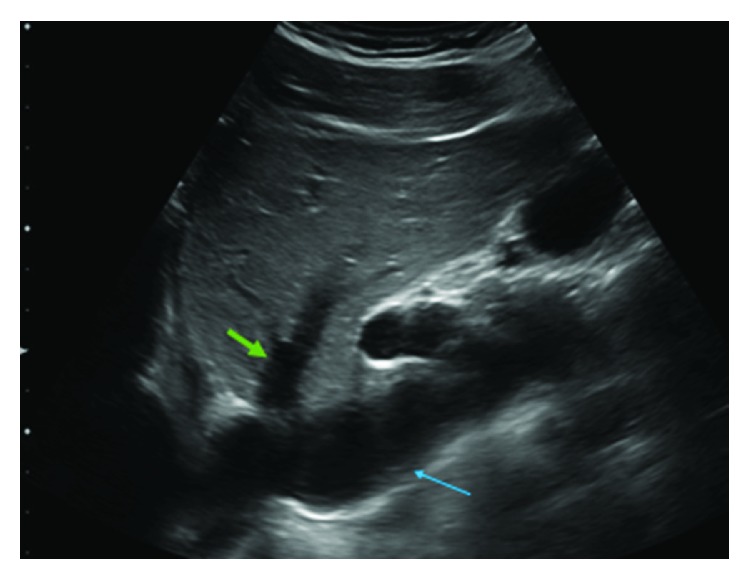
IVC on right upper quadrant ultrasound. Visualization of the hepatic vein dilatation (green) as well as the IVC dilatation (blue). Also visualized is the right atrium in the left lower corner.
